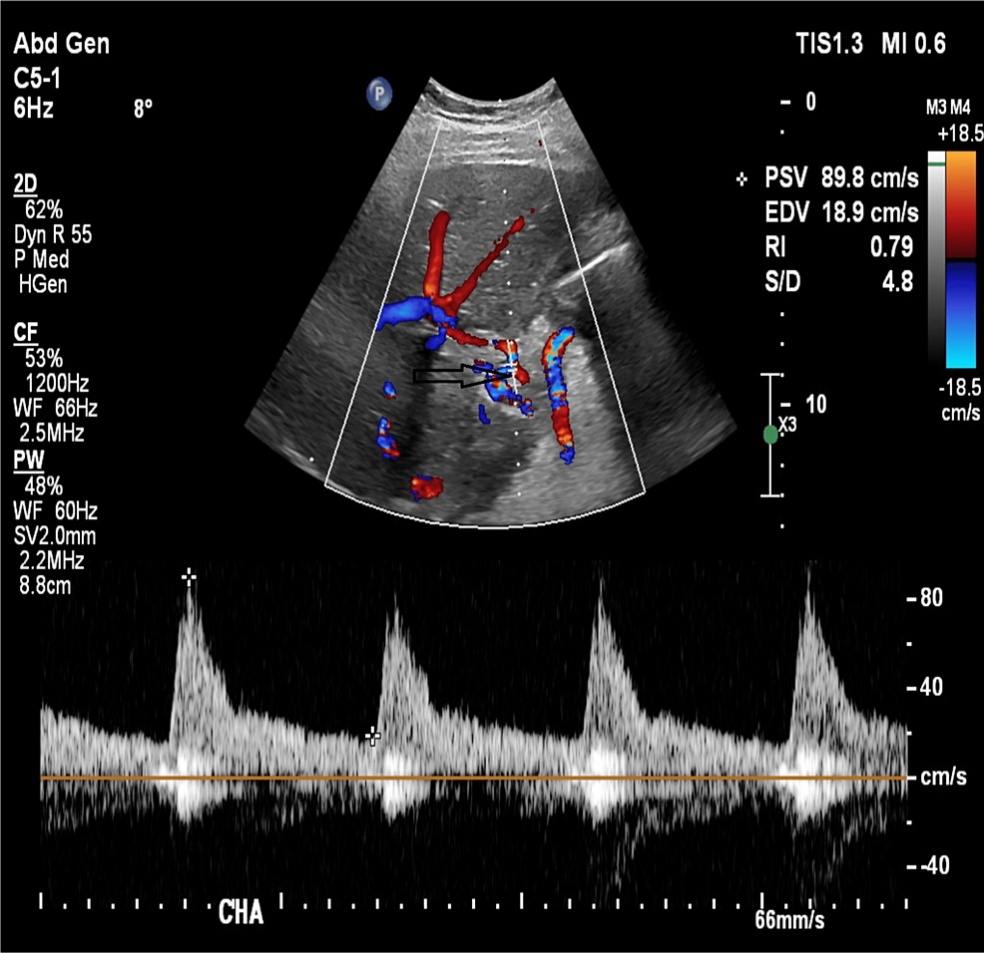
Doppler Examination of the liver on the first postoperative day.
